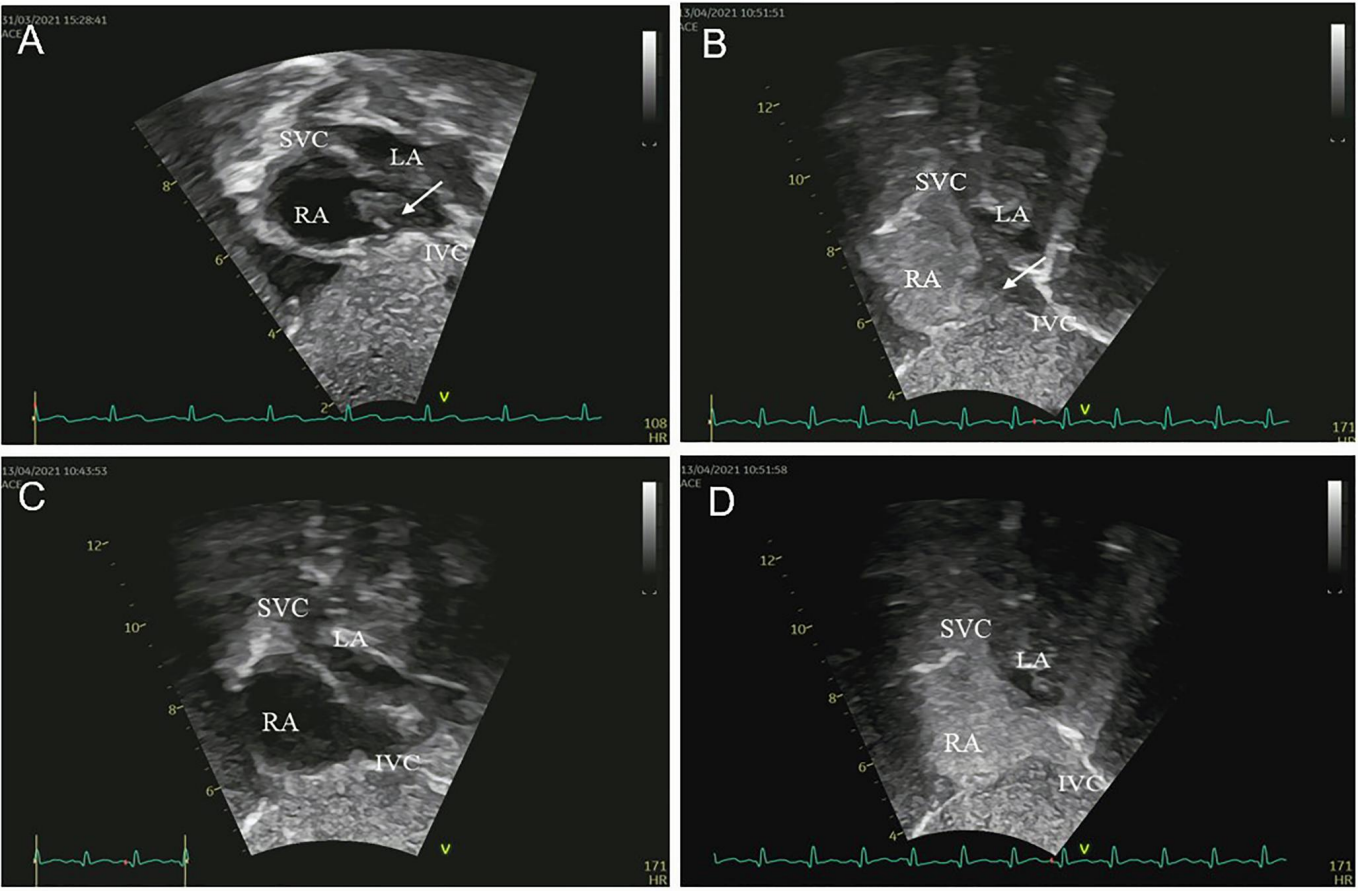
Pre and post‐treatment ultrasound images. (A) Pretreatment ultrasound 2D image. (B) Pretreatment cTTE image. (C) Post‐treatment ultrasound 2D image. (D) Post‐treatment cTTE image. cTTE, contrast transthoracic echocardiography; LA, left atrium; RA, right atrium; SVC, superior vena cava.
